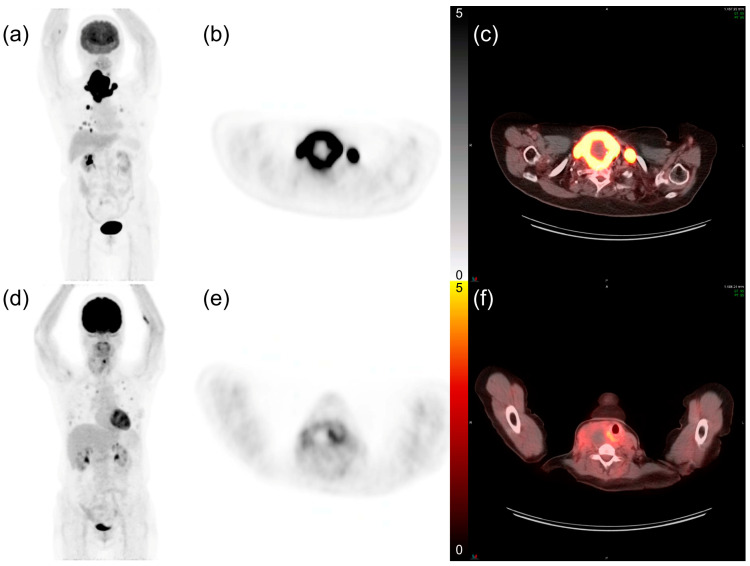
Representative ^18^fluorodeoxyglucose ([^18^F]FDG) positron emission tomography (PET)/computed tomography (CT) images of patients with anaplastic thyroid cancer and lung metastases. (a) The maximum intensity projection image of a 74-year-old female patient. (b) PET axial and (c) fusion axial images show intense FDG uptake in the thyroid tumor. The maximum standardized uptake value (SUVmax) was 35.98, and the patient expired 2.1 months after diagnosis. (d–f) The maximum intensity projection, PET axial, and fusion axial images of a 63-year-old female patient with a tumor SUVmax of 8.81. Her overall survival time was 8.1 months.
